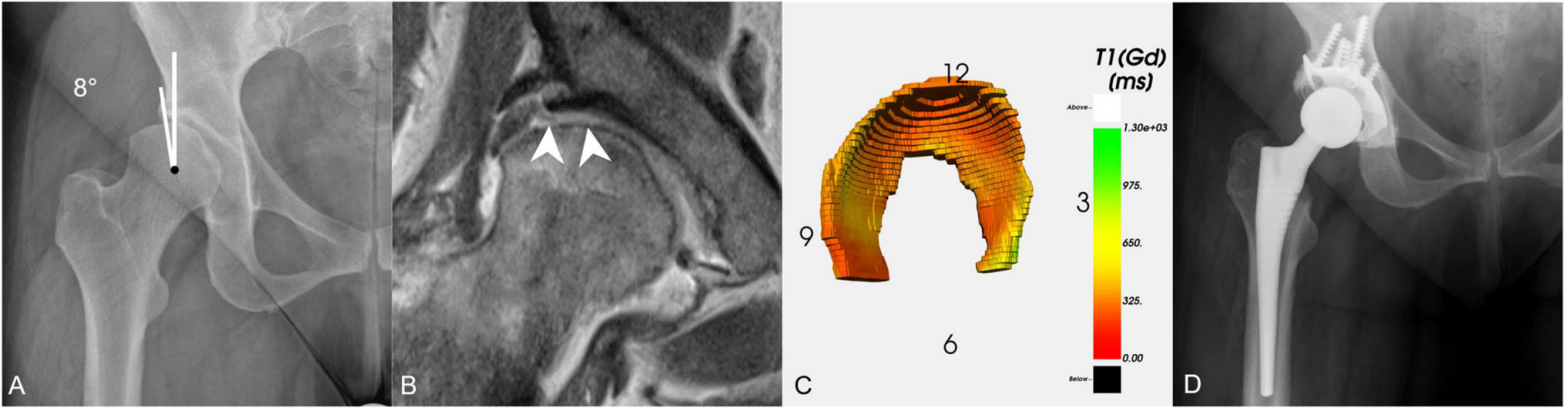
A Middle-aged woman with severe hip dysplasia (lateral centre edge angle of 8$$^\circ $$) and only mild radiographic signs of osteoarthritis. B Hip MRI showed focal femoroacetabular cartilage thinning but no obvious signs of general joint degeneration. C 3D cartilage model with colour-coded dGEMRIC indices shows generalized decreased dGEMRIC indices (overall mean 358 ms) corresponding to severe biochemical cartilage damage. D The patient underwent total hip arthroplasty
